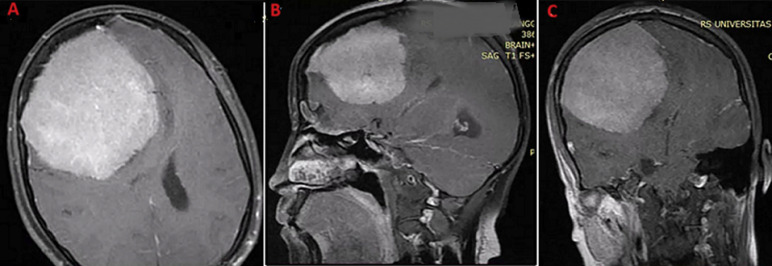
A) axial section on T1-contrast head magnetic resonance imaging showing a hyperintense lesion of giant convexity meningioma; B) sagittal; C) coronal
